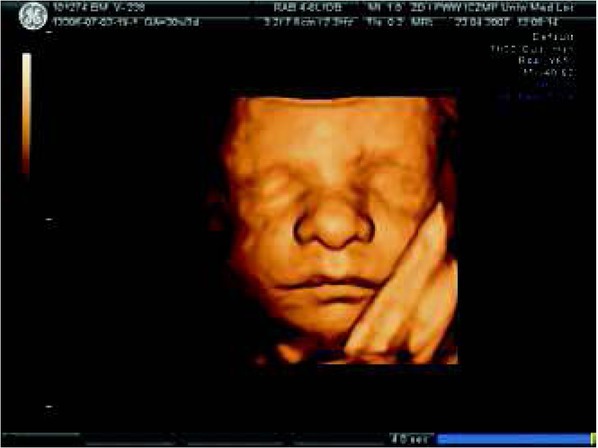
Fetal face in 3D ultrasound at the 30th week of gestation and no dysmorphic features. Patient during fetal echocardiography monitoring his haemodynamic condition due to his heart defect (since embryogenesis.) Ryc. 3. Zarysy twarzy płodu w badaniu USG w prezentacji 3D w 30 tyg. ciąży bez cech dysmorfii. Na tym etapie ciąży, wiedząc, iż u płodu powstała w okresie embriogenezy wada serca prowadzimy monitorowanie echokardiograficzne stanu hemodynamicznego pacjenta.
